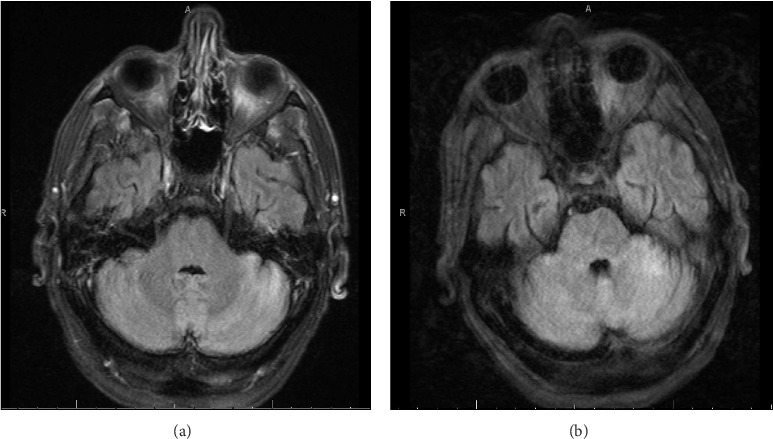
MRI images on day 6 of hospitalization. (a) T2 hyperintense signal involves the superior cerebellum and associated leptomeningeal enhancement. (b) T2 flair sequence.
